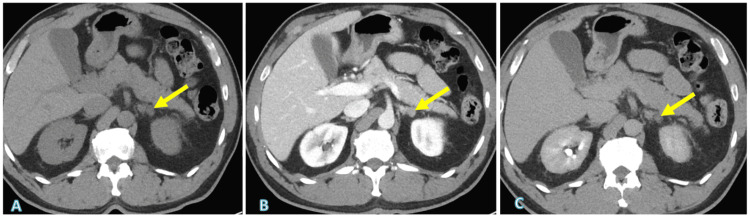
Left adrenal adenoma: axial sections from a dedicated adrenal protocol CT reveal a well-marginated left adrenal nodule (yellow arrows) with low baseline density on unenhanced imaging (A), interval enhancement following contrast administration (B), and marked washout on delayed phase images (C), in keeping with adrenal adenoma.
